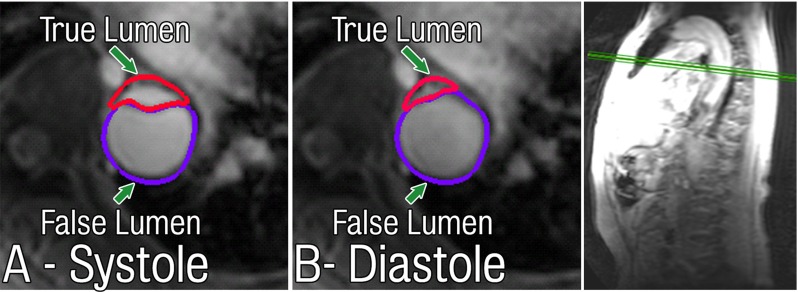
2D MRI showing distention of the dissection flap between peek systole (A) and mid-diastole (B)
